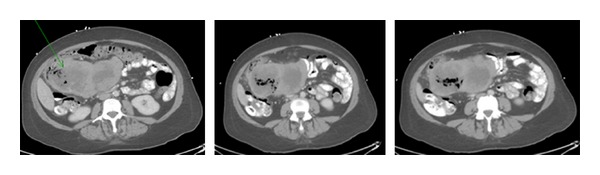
Computed tomography of the abdomen showing a retroperitoneal mesenteric mass with fistulization (communication) into the right colon.
